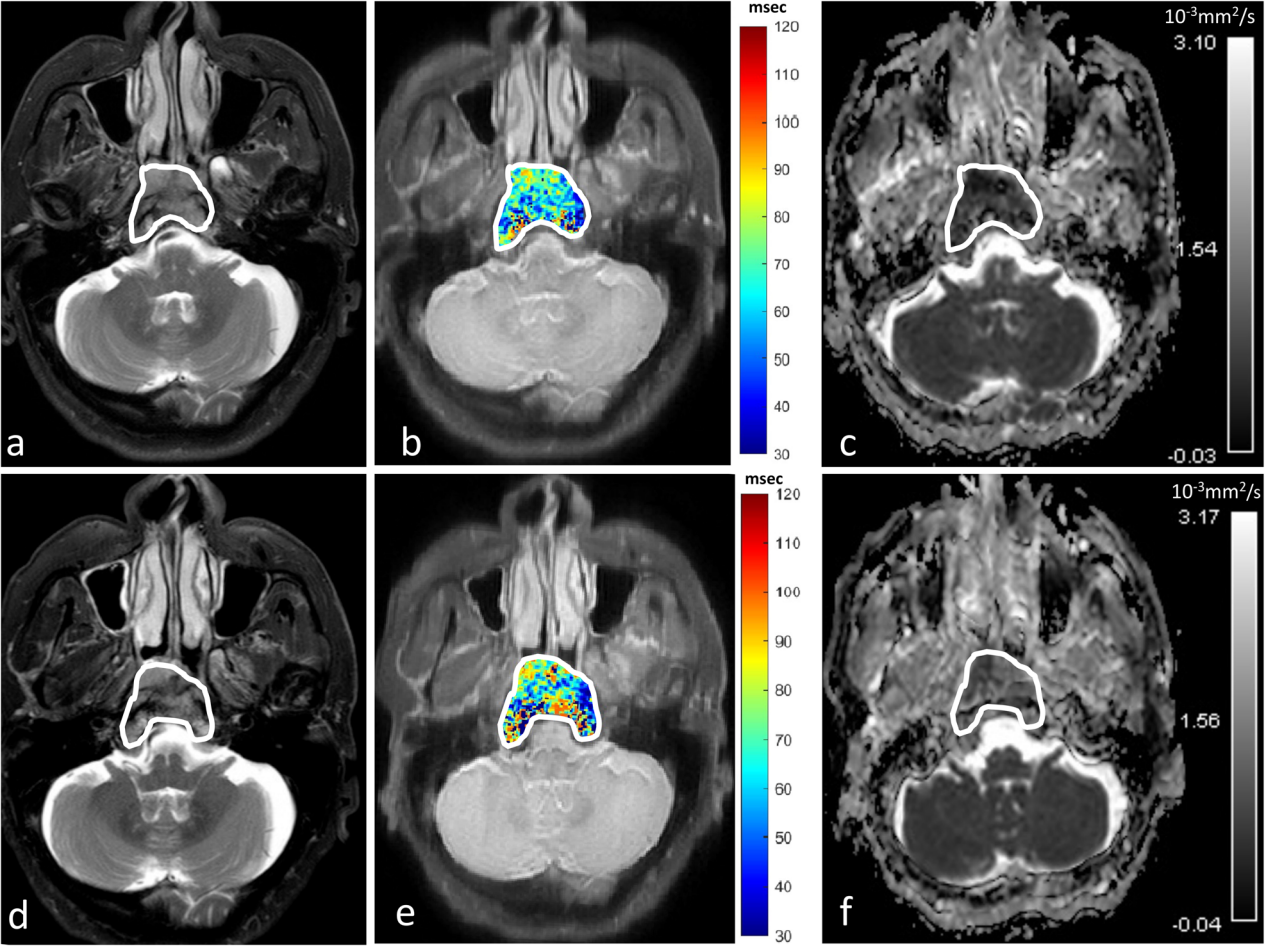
Pre-treatment (a–c) and early intra-treatment (d–f) MRIs of a patient with primary NPC that did not respond to IC and showed residual disease on biopsy after treatment. The axial images comprise T2-weighted fat-suppressed images (a, d), T1rho maps (b, e) and ADC maps (c, f). The Δ% for mean T1rho was + 6.0%, and for mean ADC was + 11.3%. Poor response to IC was predicted by a percentage increase in mean T1rho value compared to a decrease for responders (i.e. ΔT1rho% of > − 3.4%) and residual disease was predicted by a lower percentage increase in the mean ADC than expected for responders (i.e. ADC increase was ≤ + 16.8%). NPC, nasopharyngeal carcinoma; CRT, chemoradiotherapy; IC, induction chemotherapy; ADC, apparent diffusion coefficient; MRI, magnetic resonance imaging
